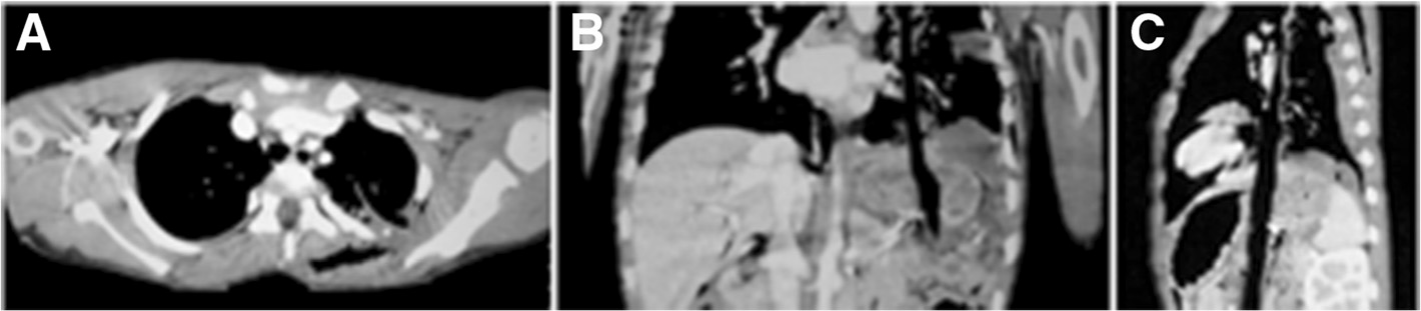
A–C. CT scan findings on ED presentation. The impaled piece of bamboo can be seen as a hollow air-containing tube extending vertically in the left abdomen and thorax.
